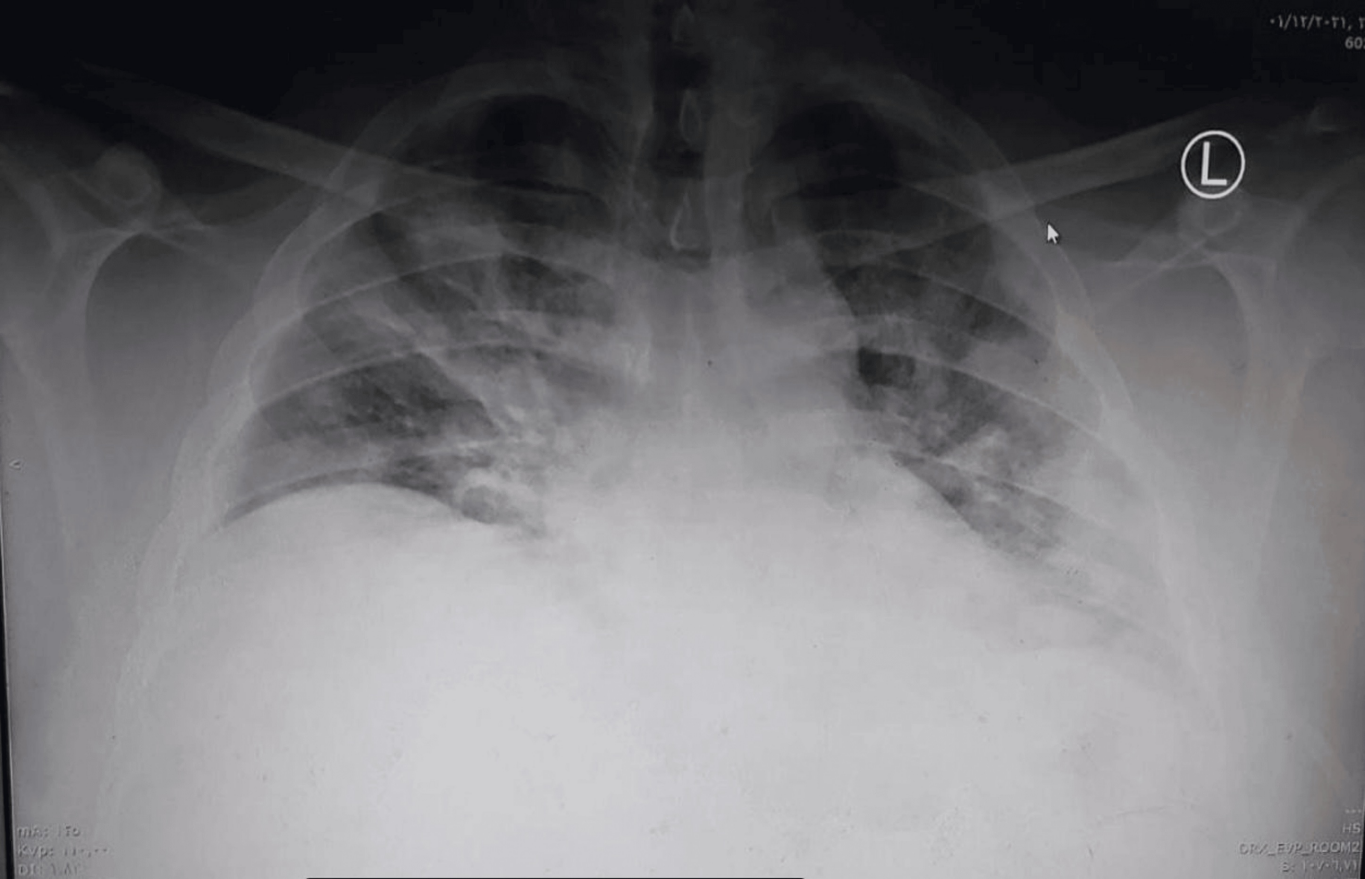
Chest X-ray on admission (Case 2)
